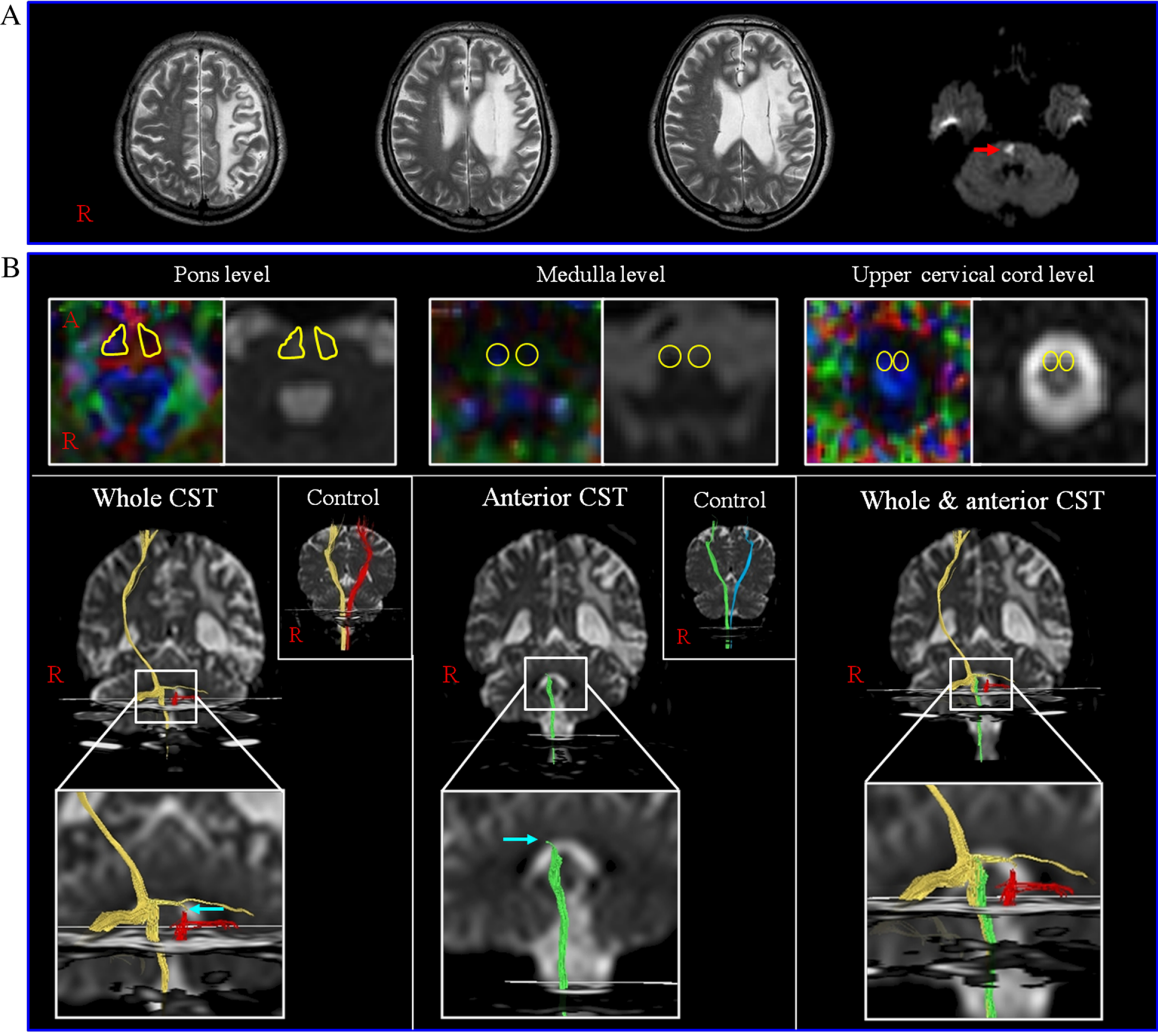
Brain MRI and results of diffusion tensor tractography. A) Brain MRI showing an old infarct in the left middle cerebral artery territory and a new infarct in the right pontine basis (arrow). B) Regions of interest for the whole and anterior corticospinal tract (CST)(yellow-lined circle) and results of diffusion tensor tractography. The whole CST and the anterior CST were constructed in the patient and a normal control subject (yellow: right whole CST, red: left whole CST, green: right anterior CST, blue: left anterior CST).
